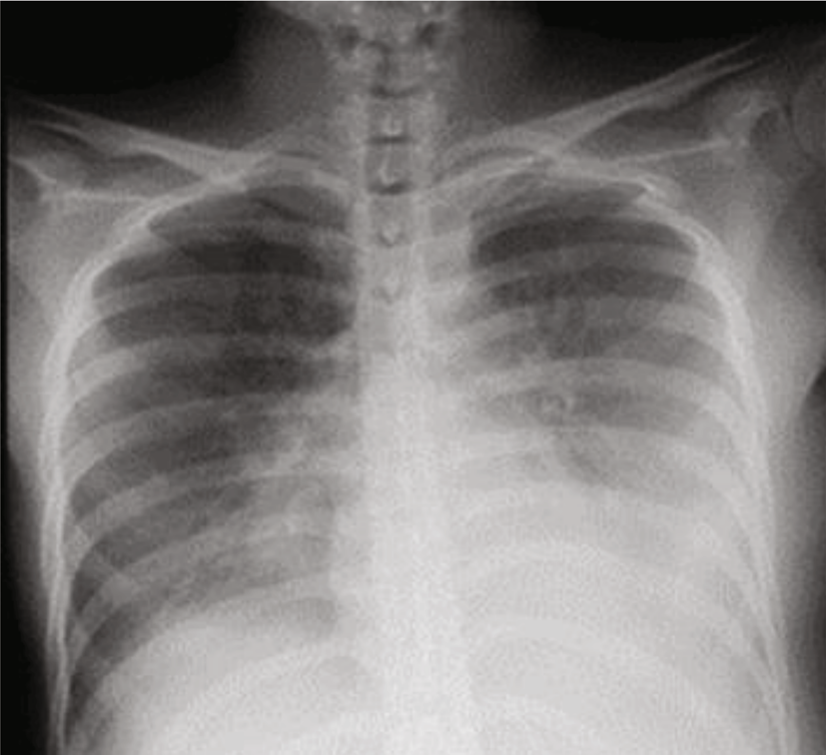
Chest radiograph at postpartum day 2 shows bilateral pleural effusion.
